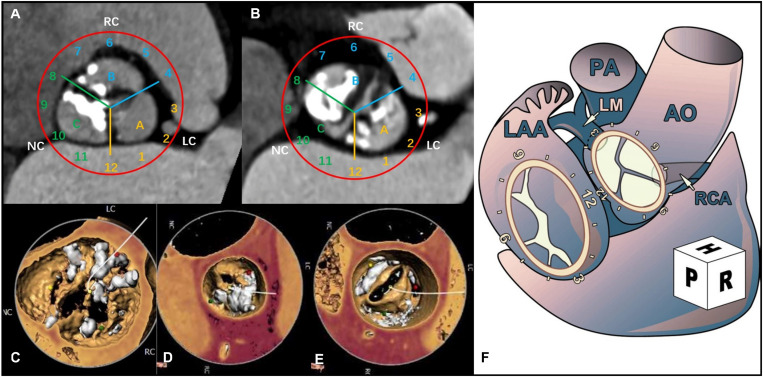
Computerized tomography angiography (CTA) shows the distribution of aortic valve calcification using a surgeon’s view clock-face model. (A) The CTA cross section shows the distribution of the tricuspid aortic valve (TAV) calcification. (B) The CTA cross section shows the distribution of the bicuspid aortic valve (BAV) calcification. (C) 3-Dimensional (3D) view of the CTA image shows the eccentric calcification of the aortic valve in region A. (D) 3D view of the CTA image shows eccentric calcification in region B. (E) 3D view of the CTA image shows eccentric calcification in region C. (F) Surgical view of the left atrium (Ruiz et al., 2011).
